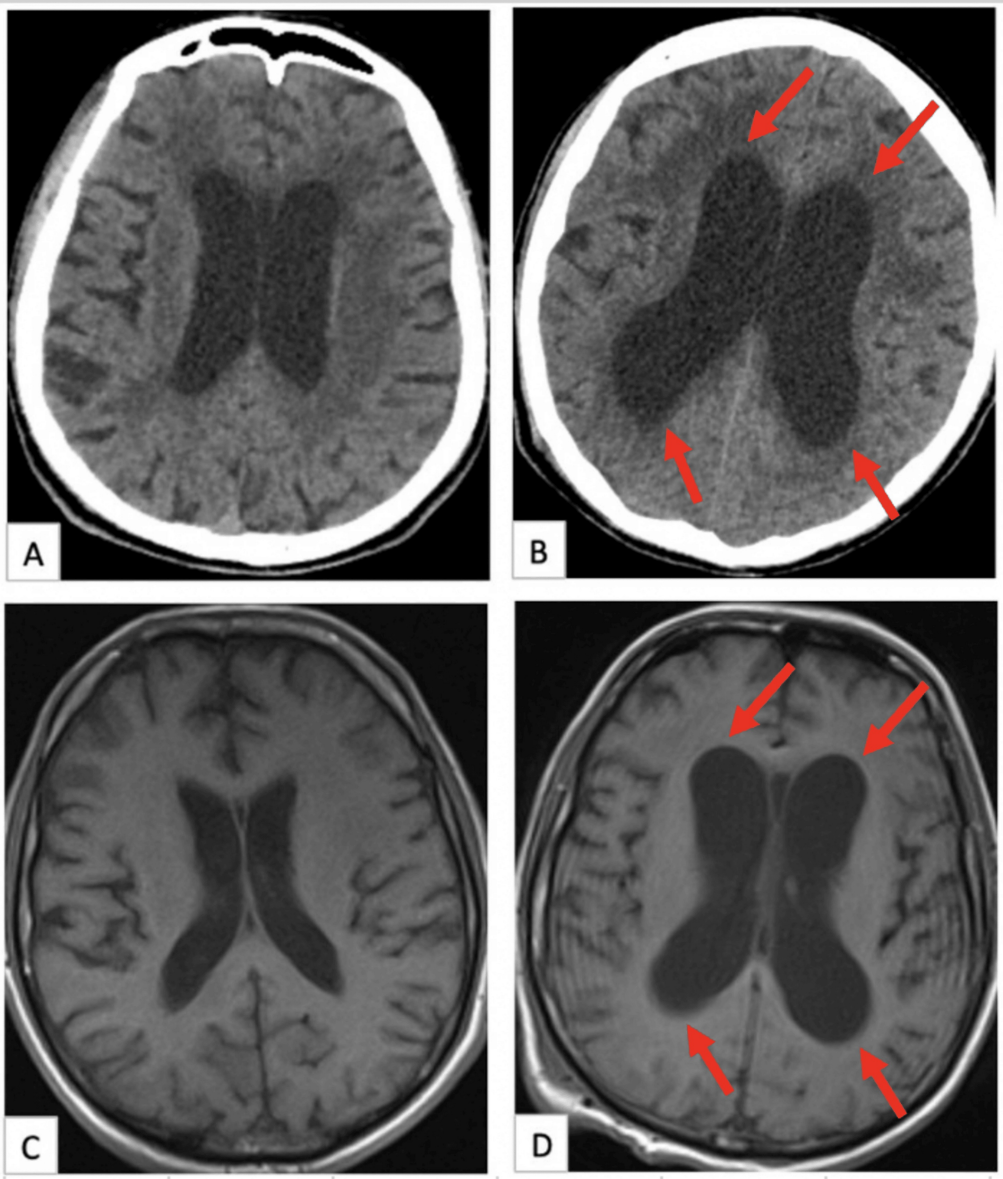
Cranial images of the patient A: Cranial CT before surgery, B: Cranial CT showing the development of hydrocephalus during follow-up, C: Cranial MRI before surgery, and D: Cranial MRI showing the development of hydrocephalus during follow-up. Red arrows show that ventricle sizes are more dilated.
